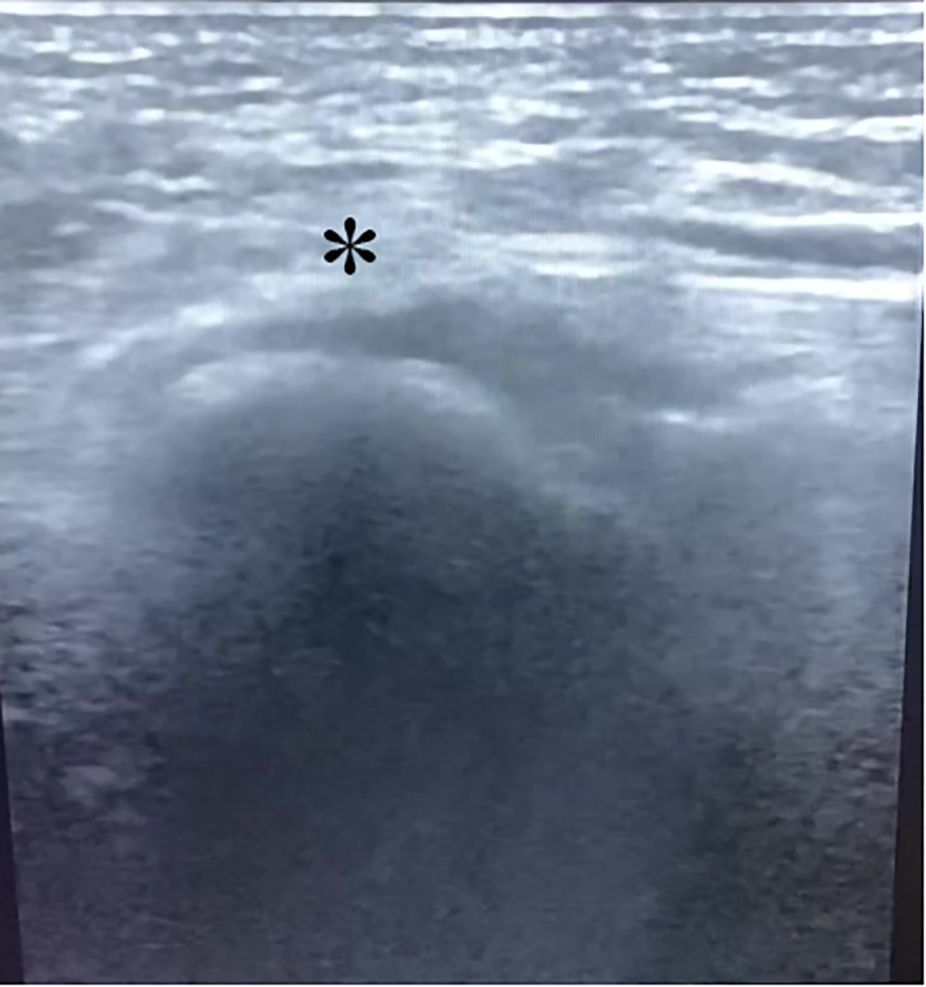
The asterisk indicated the presence of FHL tendon in the malleolar canal by the dynamic ultrasound.
